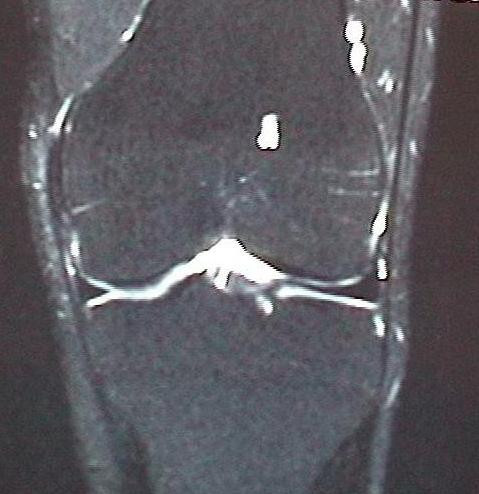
Complete normalization of the MRI signal pattern after 6 months of conservative treatment.
